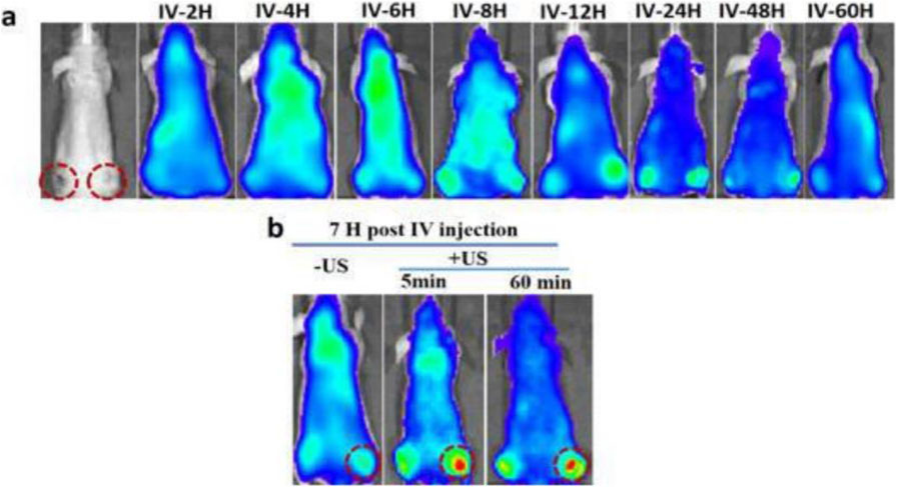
In vivo* NIR flourescent imaging. (a) The distribution of drug in tumor after I.V. injection. (b) Ultrasound comnbined with microbubbles enhanced the fluorescence of Pyro-liposome, facilitating NIR and photoacoustic imaging and sonochemical therapeutics. A dual tumor model was used, with a tumor on the right flank was irradiated and the other was non-irradiated
